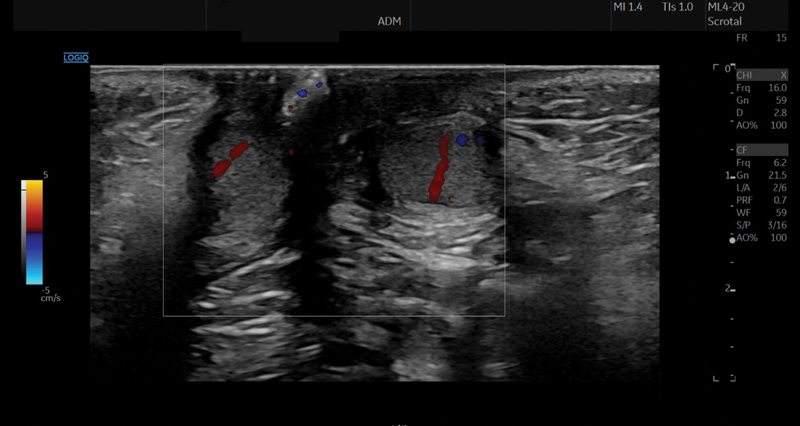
Doppler sonography 12 months postoperatively.
